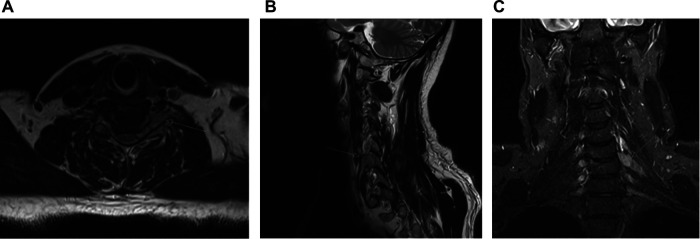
Axial (A), sagittal (B), and coronal (C) post-contrast T1 MRI showing extensive intraspinal, extradural tumor, including extension into the left C5/6 neural exit foramen.
